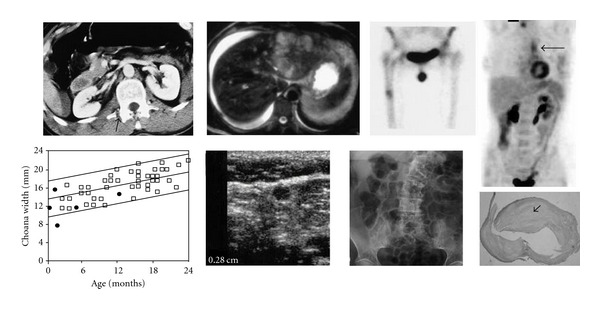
Sample images of 8 medical modalities. From left to right and top to bottom, the images are CT, MR, NM, PET, GX, US, XR, and PX, respectively.
